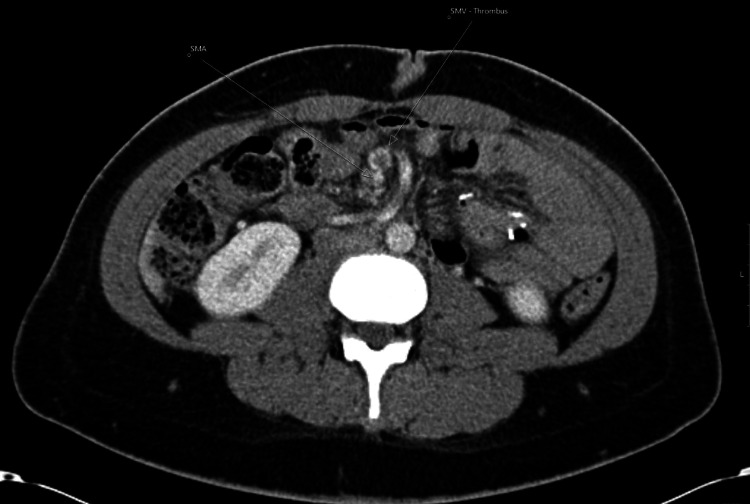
CT scan demonstrating SMV containing thrombus (arrow) and normal SMA (arrow) CT: computed tomography, SMV: superior mesenteric vein, SMA: superior mesenteric artery
